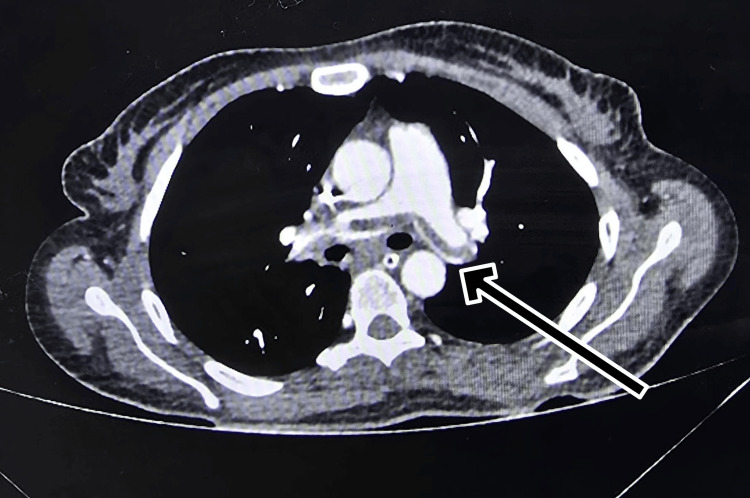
CT pulmonary angiography of the chest showing acute pulmonary embolism involving the bilateral main pulmonary arteries.
